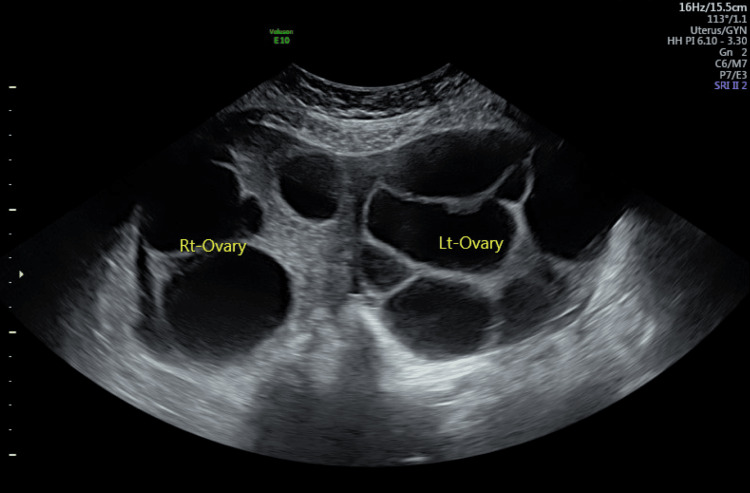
Ultrasound showing enlarged ovaries with cysts when she was admitted with ovarian hyperstimulation syndrome Rt Ovary: Right ovary, Lt Ovary: Left Ovary
